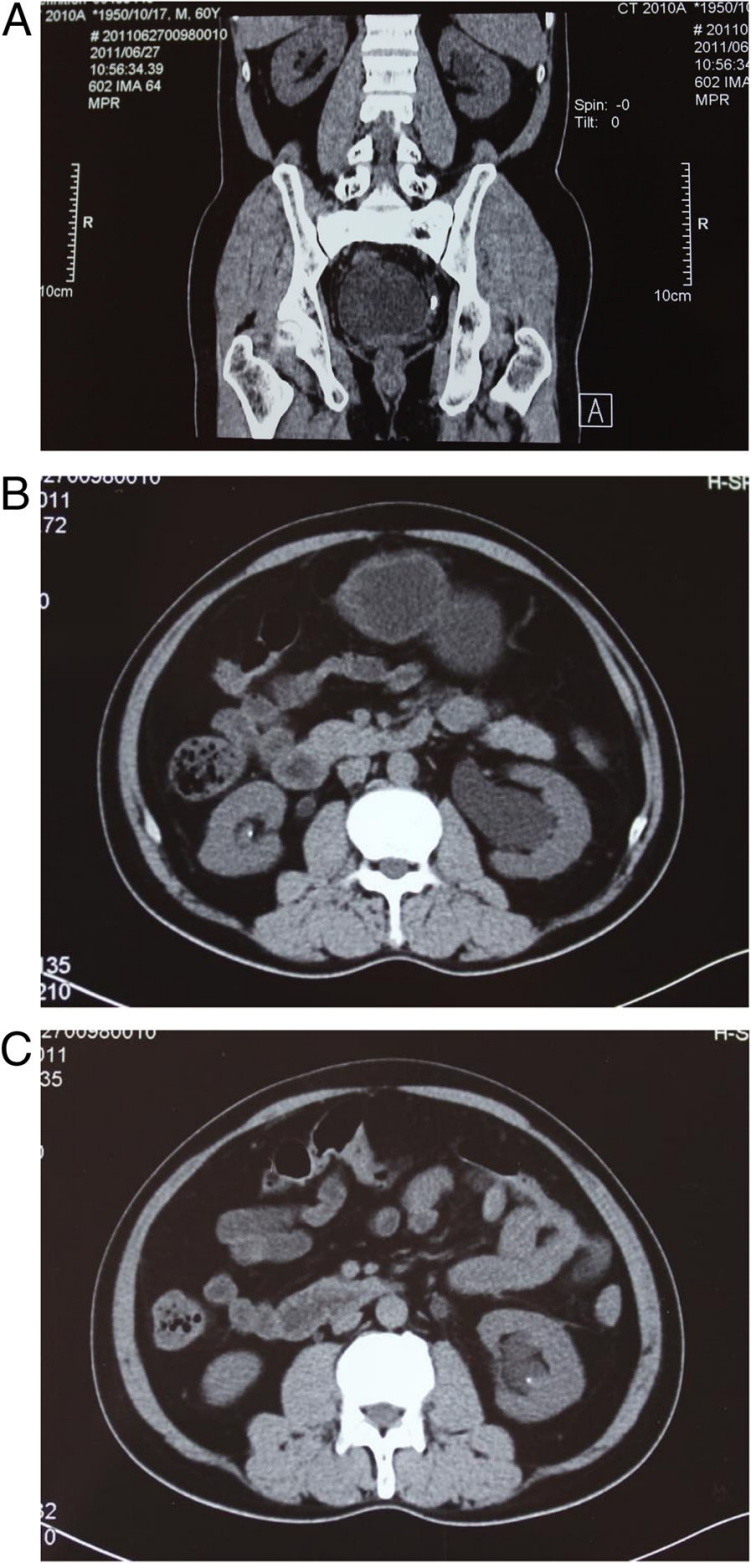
CT imagines. a: left ureteral stone combined with left hydronephrosis. b: a stone in the right kidney. c: a stone in the left kidney
